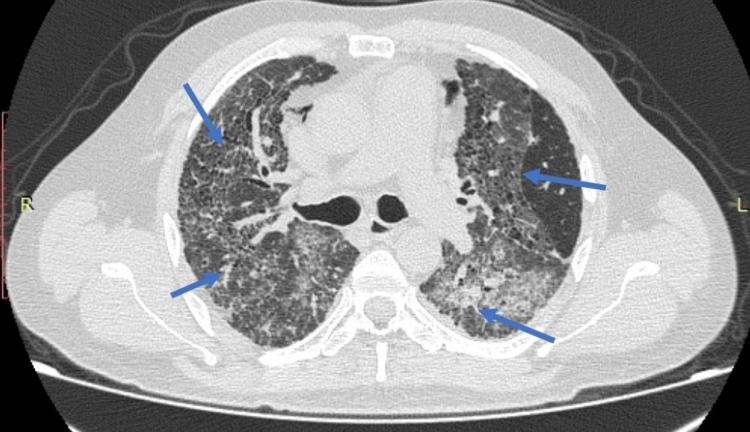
CT chest showing interstitial infiltrates in both lung fields. CT: Computed tomography
